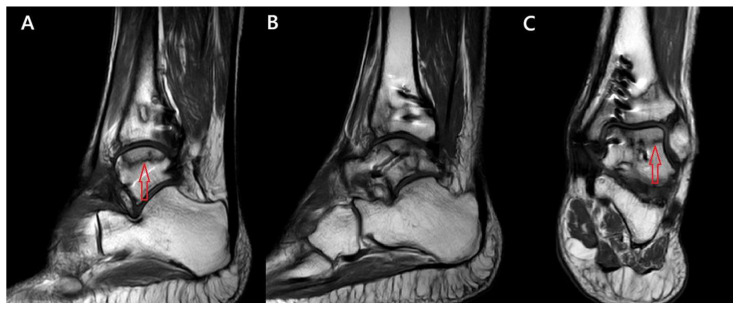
(A,B) Sagittal and (C) coronal MRI planes, revealing the early signs of talar AVN. The red arrows indicate the regions of AVN of the talus.
